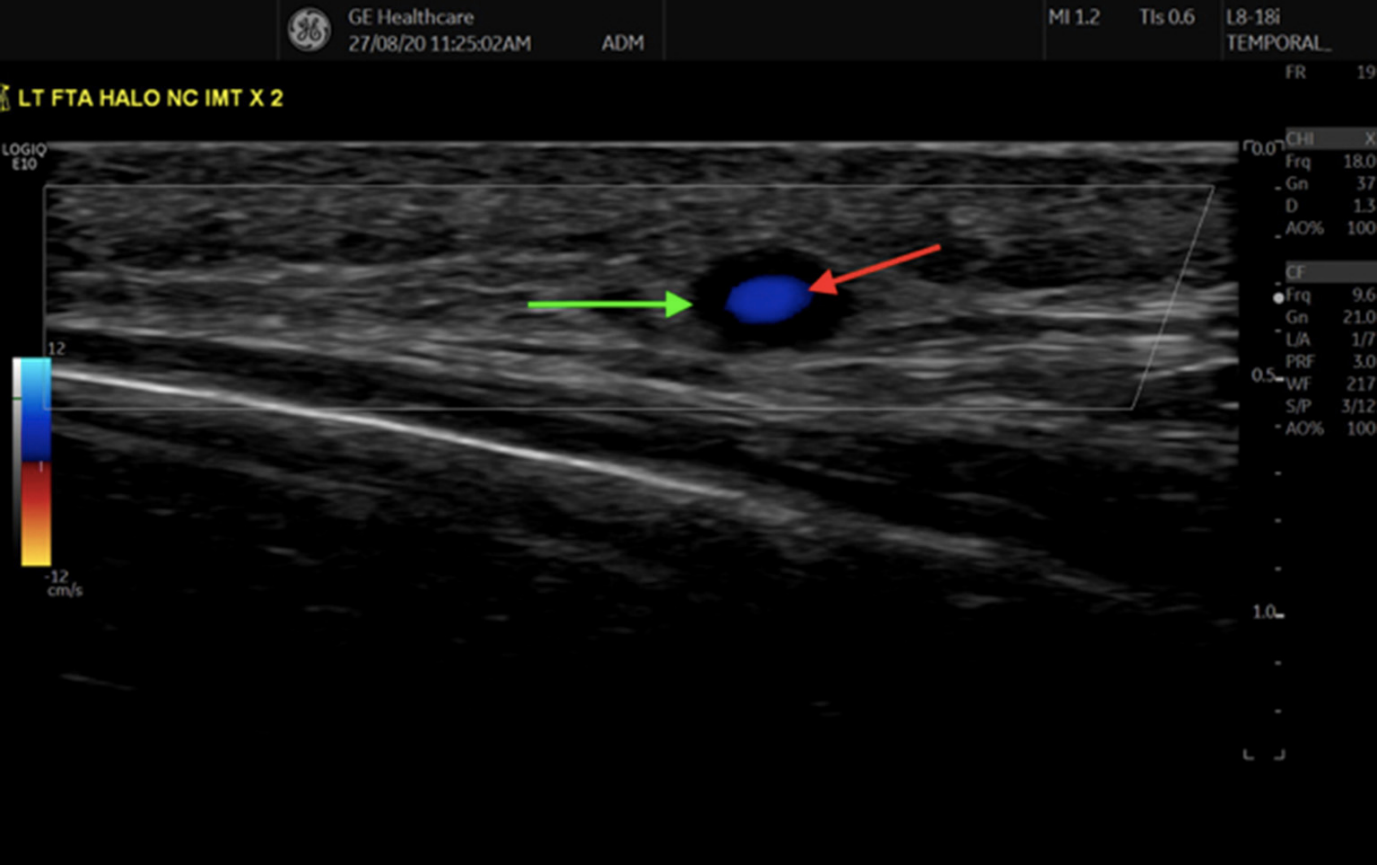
Transverse view of the frontal branch of the Superficial Temporal Artery, demonstrating a halo sign, as indicated by the anechoic region (green arrow) surrounding the inner Doppler (red arrow) signal.
